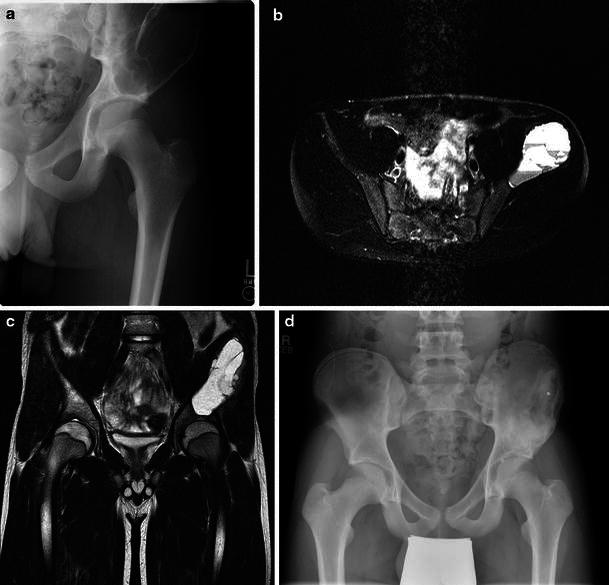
Aneurysmal bone cyst (ABC) of the left iliac wing adjacent to the acetabulum in a 14-year-old-male that presented with worsening left hip pain in the past 8 months. a Anteroposterior radiograph reveals a very large expansile, osteolytic lesion of the left ilium extending into the superior acetabulum. b Axial T2-weighted magnetic resonance imaging (MRI) reveals multiple fluid levels characteristic of an aneurismal bone cyst. c Coronal T2-weighted MRI demonstrating extension into the supra-acetabular region with no involvement of the joint. d Anteroposterior pelvic radiograph 2 years postoperative reveals no signs of local recurrence and complete bone healing of the entire cystic area. The patient at most recent follow-up was very active and had returned to high school baseball, wrestling, and football without limitation
